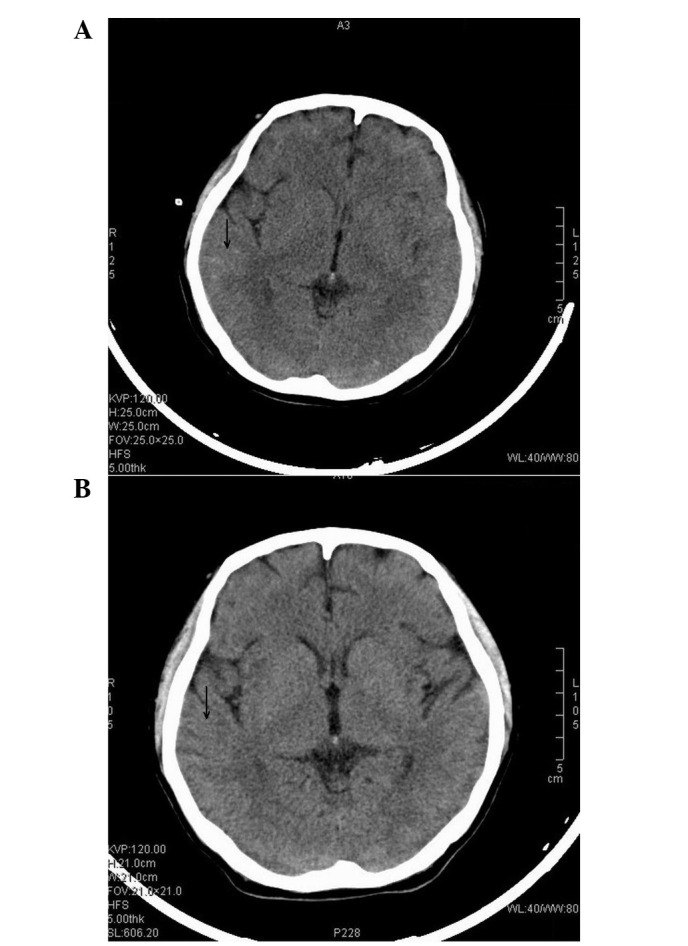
Cranial computed tomography film of Case 1. (A) High-density patchy areas were observed following multiple intracranial hemorrhages. (B) Improvement in hemorrhage following treatment. The arrows in the images indicate the place of bleeding.
